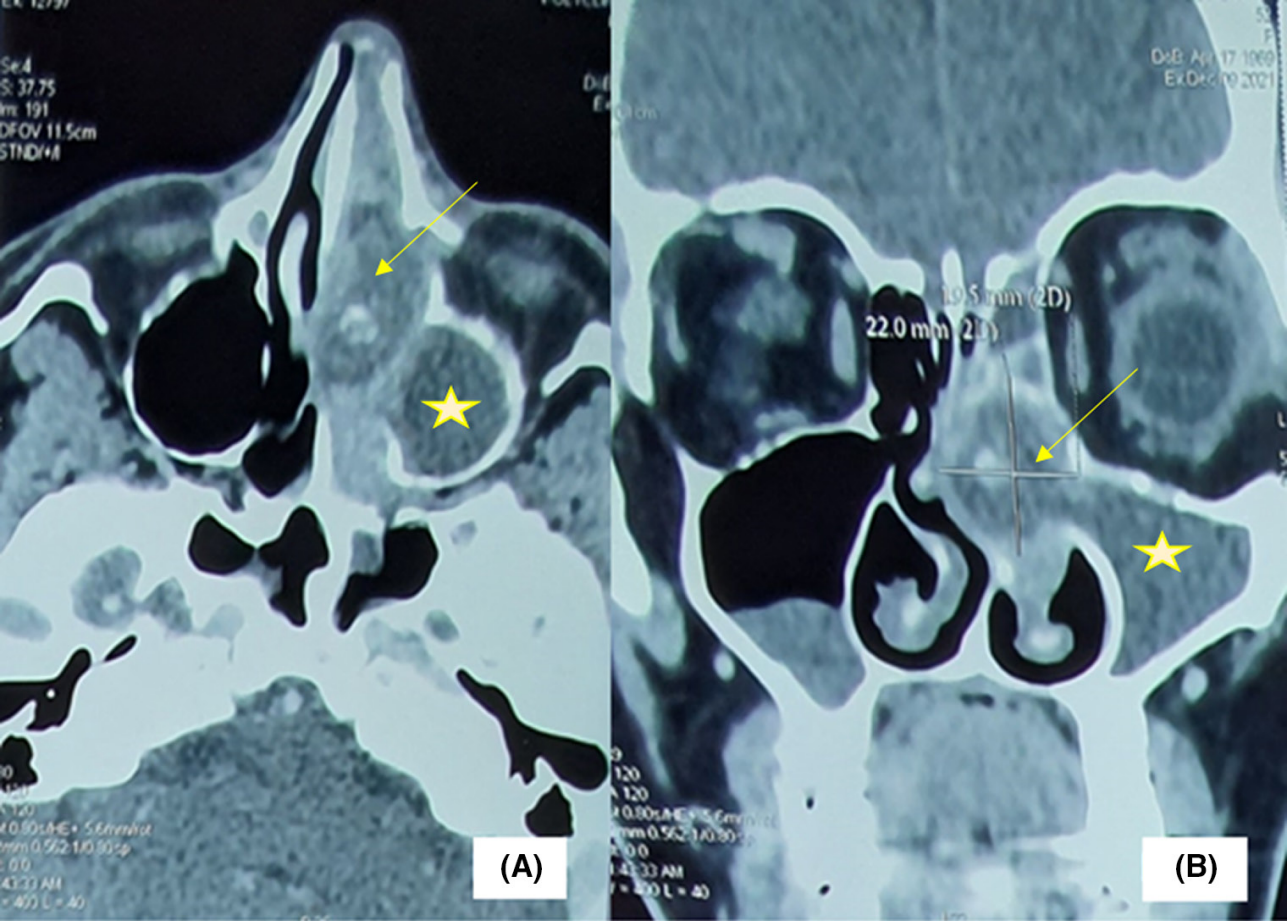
Axial (A) and coronal (B) paranasal sinus computed tomography scan showing a heterogeneous left‐sided nasal mass attached to the medial turbinate (arrow) with an ipsilateral maxillary and ethmoid sinuses opacities (stars)
